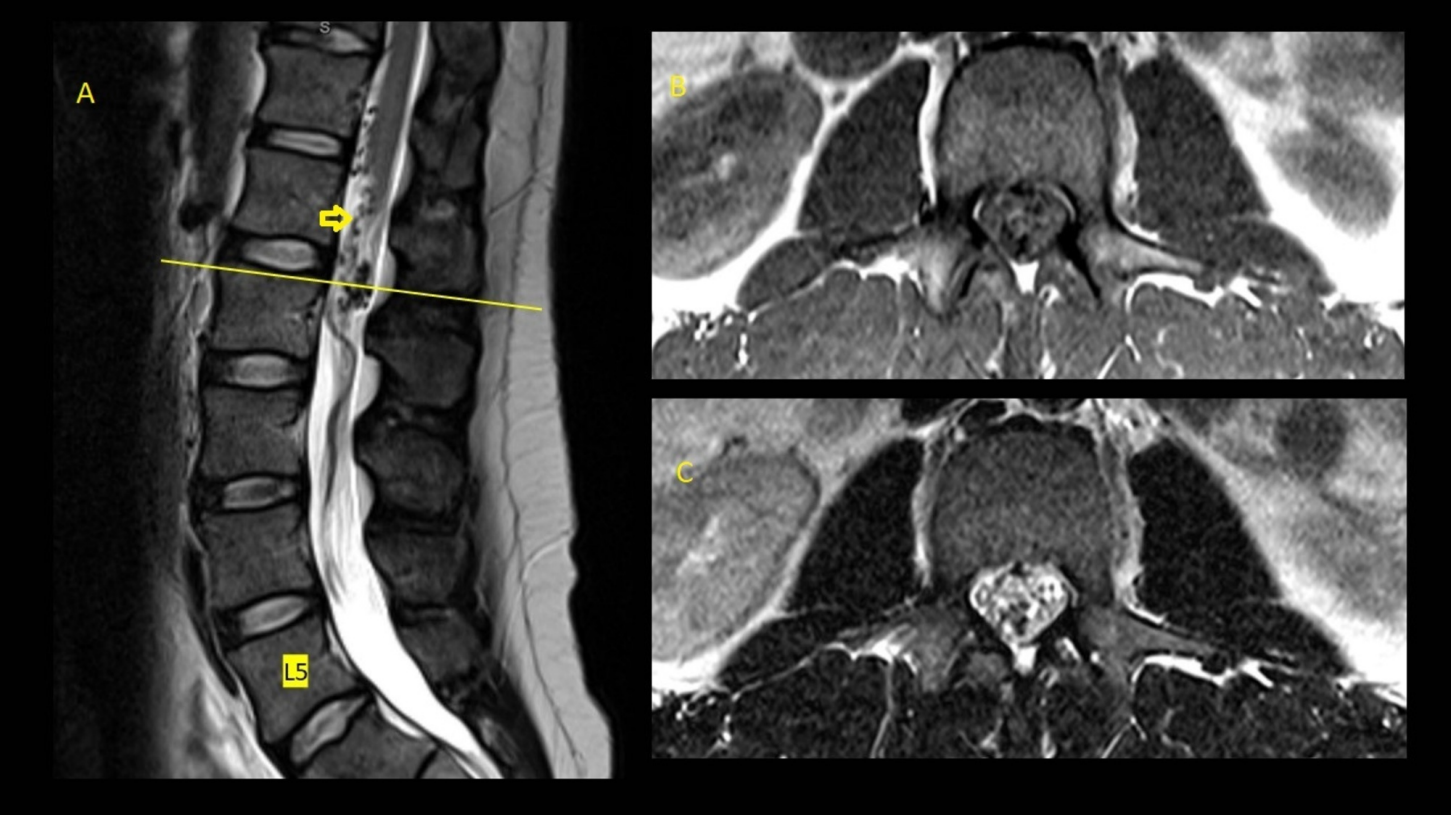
Sagittal T2 (A) and axial T1 (B) and T2 (C) magnetic resonance imaging showing the conus arteriovenous malformation with tortuous vessels surrounding the filum terminale. The yellow arrow points at the junction point between the filum and the vascular malformation.
